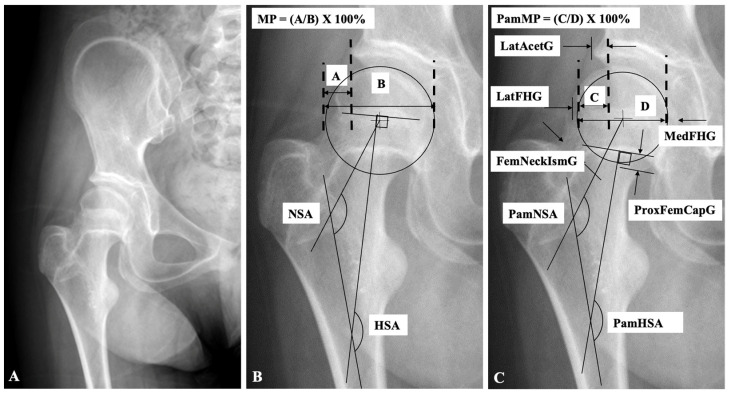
These pelvis and proximal femur radiographs are from a 12.5-year-old girl with cerebral palsy, Gross Motor Function Classification System level V, treated with 8 cycles of pamidronate between ages 8.2 and 11.3 years. The visible dense lines from the pamidronate therapy outline the border of the bone when she started the treatment at age 8.2 years. (A) Measurements of her bone shape and hip status at the time of the current radiograph based on measurements of the bone shape. (B) Measurements of her bone shape and hip status based on measurements of the dense pamidronate lines. (C) FemNeckIsmG, femoral neck isthmus growth; HSA, head–shaft angle; LatAcetG, lateral acetabular growth; LatFHG, lateral femoral head growth; MedFHG, medial femoral head growth; MP, migration percentage based on the border of the bone (A/B × 100%); NSA, neck–shaft angle; PamHSA, head–shaft angle based on pamidronate bands; PamMP, migration percentage based on pamidronate bands (C/D × 100%); PamNSA, neck–shaft angle based on pamidronate bands; ProxFemCapG, proximal femoral capital growth.
